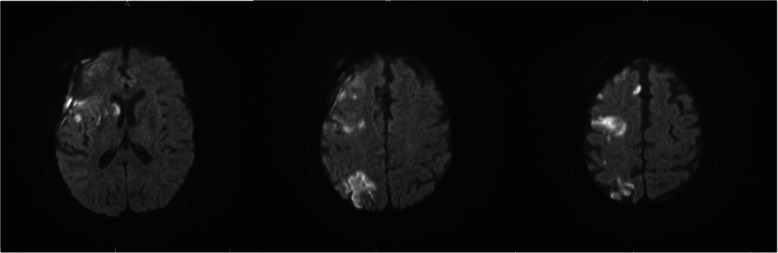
Diffusion weighted imaging sequence of MRI brain on Day-1. Acute ischemic infarct involving the right hemisphere is shown
